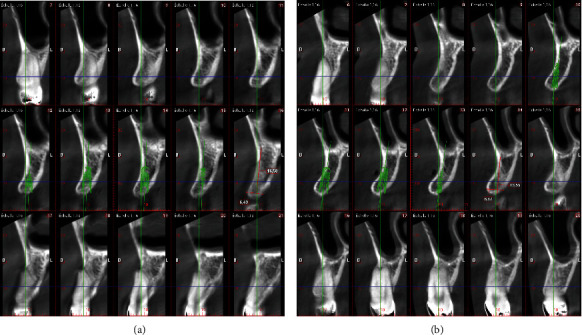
Sagittal views from the computed tomography (a) on the region of tooth number 6 with the implant prefiguration (blue) and (b) on the region of tooth number 11.
